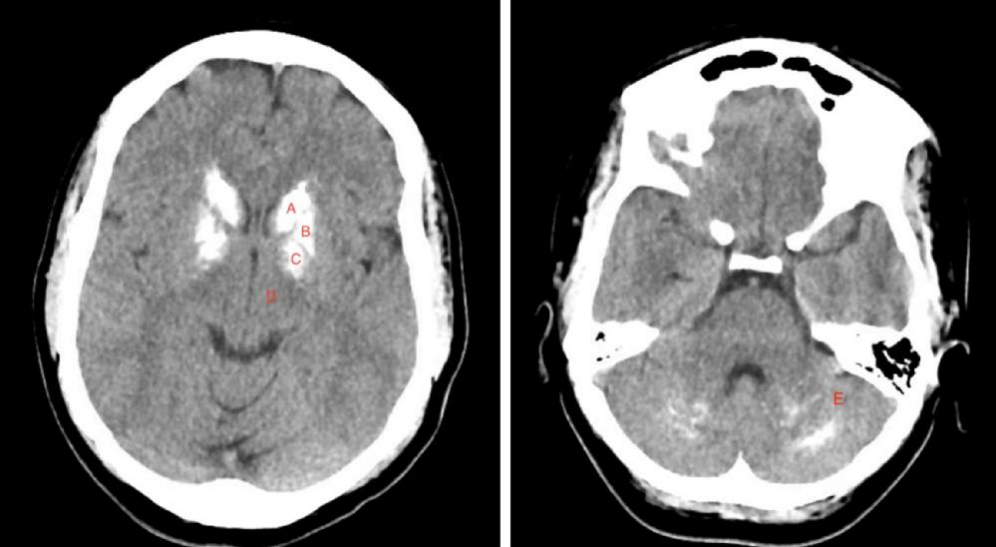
Noncontrast axial computed tomography images of the head showed calcifications in the bilateral basal ganglia, including caudate nucleus (A), putamen (B), and globus pallidus (C). The thalamus (D) appeared normal. The dentate nuclei (E) were also calcified bilaterally.
